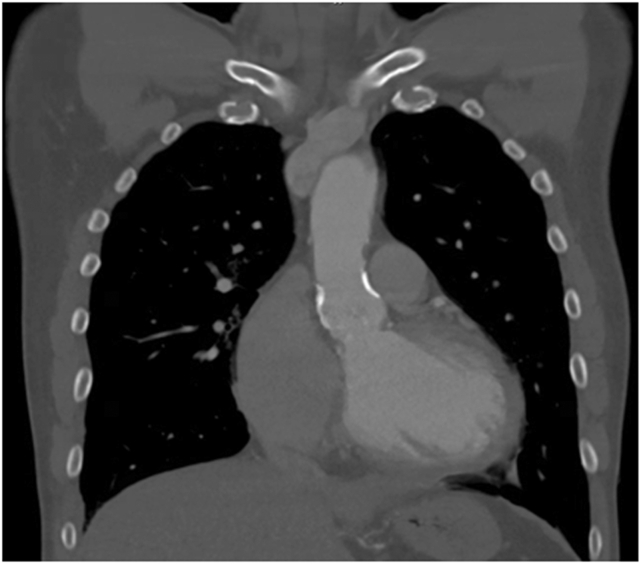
Preoperative CT scan. Coronal imaging showing calcification of the aortic root and proximal ascending aorta. *CT*, Computed tomography.
